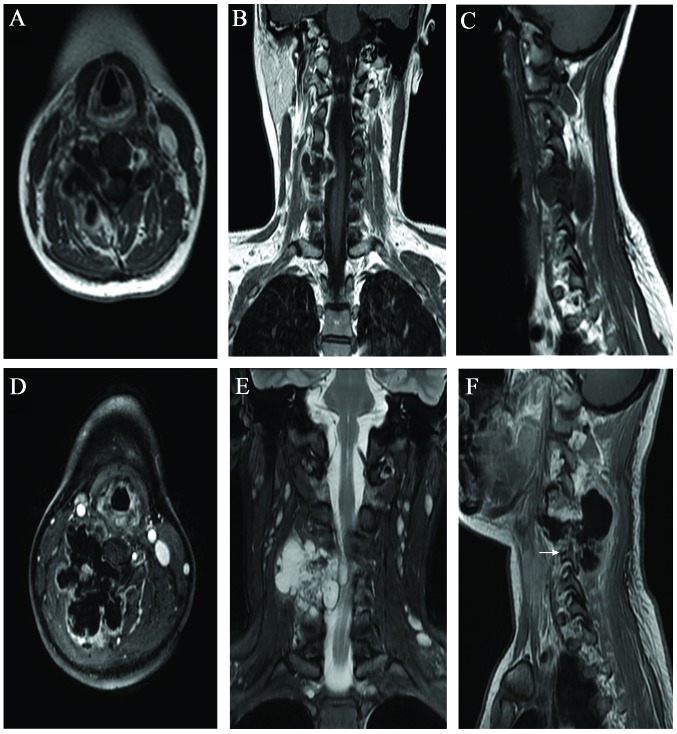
(A) Axial, (B) coronal and (C) sagittal T1-weighted images captured 3 years prior to the palpable mass of the cervical spine. (D) The coronal view, Gd-DTPA-enhanced image, showing soft-tissue expansion and the destruction of the adjacent normal tissues, including the right side of the processus transversus, lamina and spinous process. (E) The fat-suppressed image discloses a tumor lesion, and compression of the right side of the spinal cord. (F) The sagittal T1-weighted image demonstrates that the nerve root was being squeezed (white arrow). Gd-DTPA, gadopentetic acid with diethylenetriaminepentacetate.
